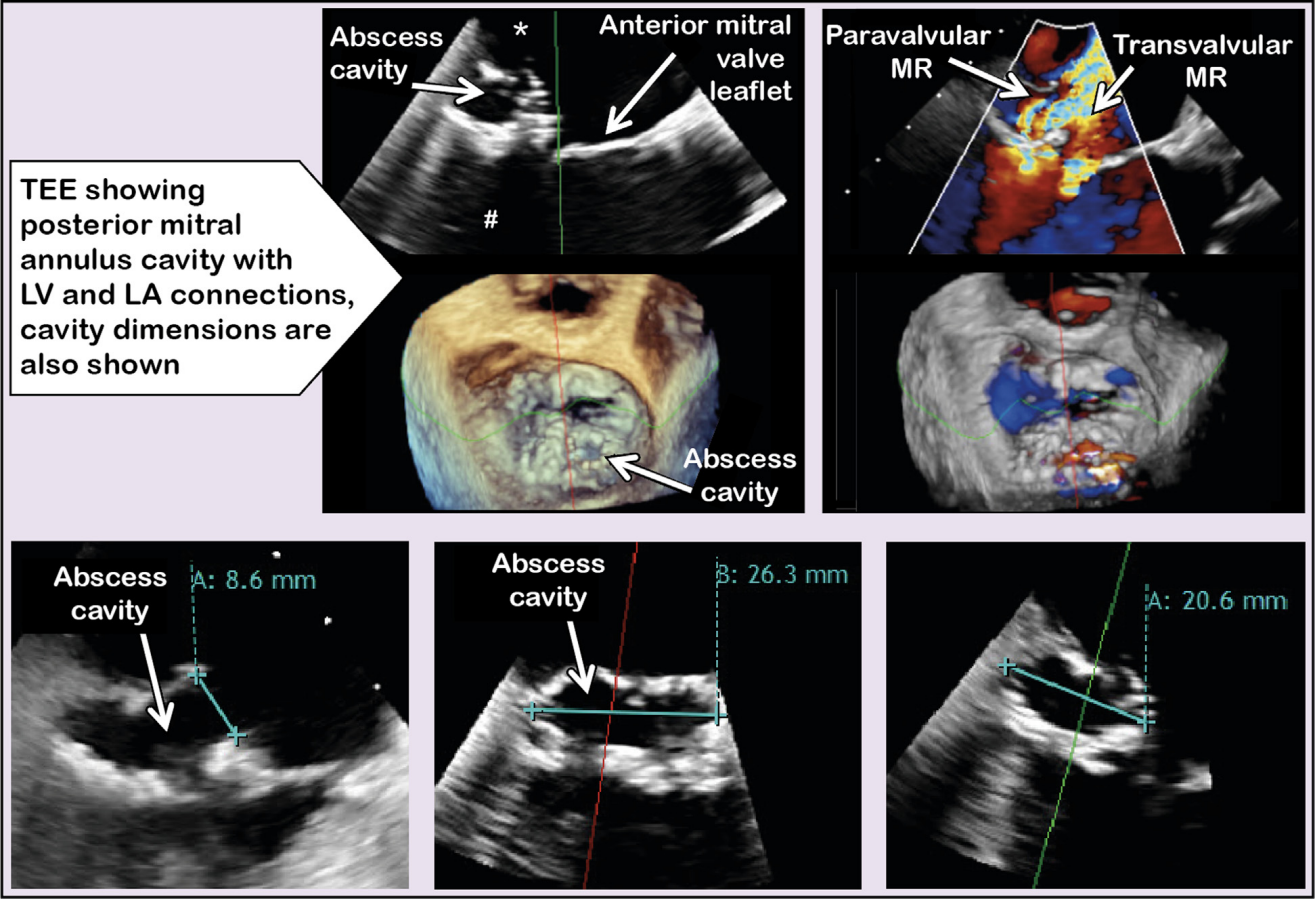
TEE Before Mitral Valve Intervention Showing Transvalvular and Paravalvular MR and a Posterior Mitral Annular Cavity (Dimensions Shown) With LV and LA Connections Asterisk denotes inflow in the left atrium (LA), and pound sign denotes outflow in the left ventricle (LV). MR = mitral regurgitation; TEE = transesophageal echocardiography.
